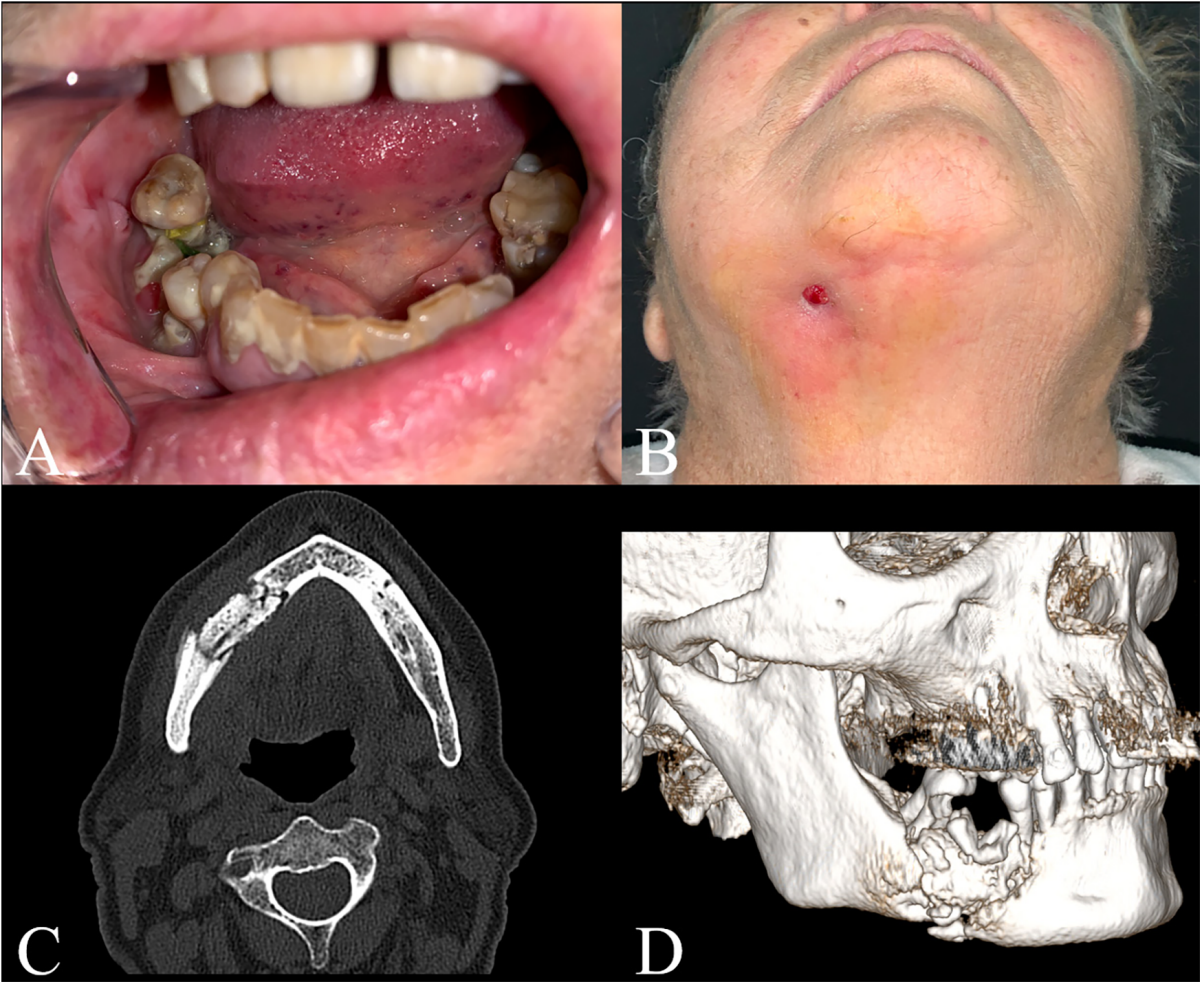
Patients’ clinical examination and preoperative CT scan. (A) Intraoral view; (B) Submentovertex view; (C) Axial view; (D) 3D reconstruction.
